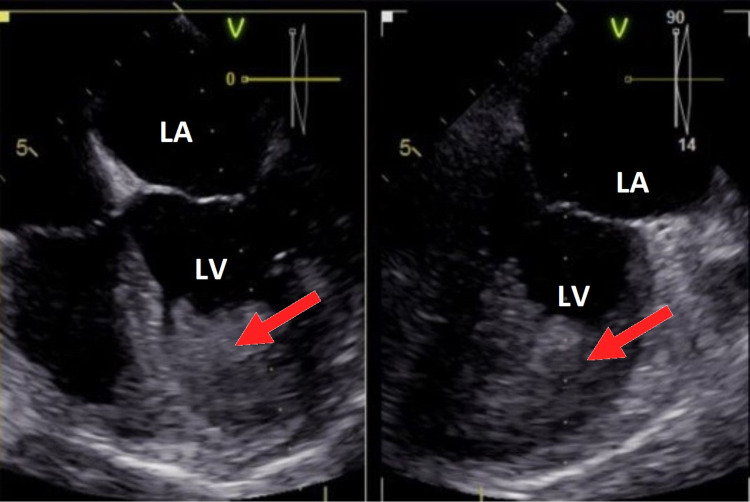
A TEE revealing a gigantic LV mass. TEE, transesophageal echocardiogram; LV, left ventricle; LA, left atrium
